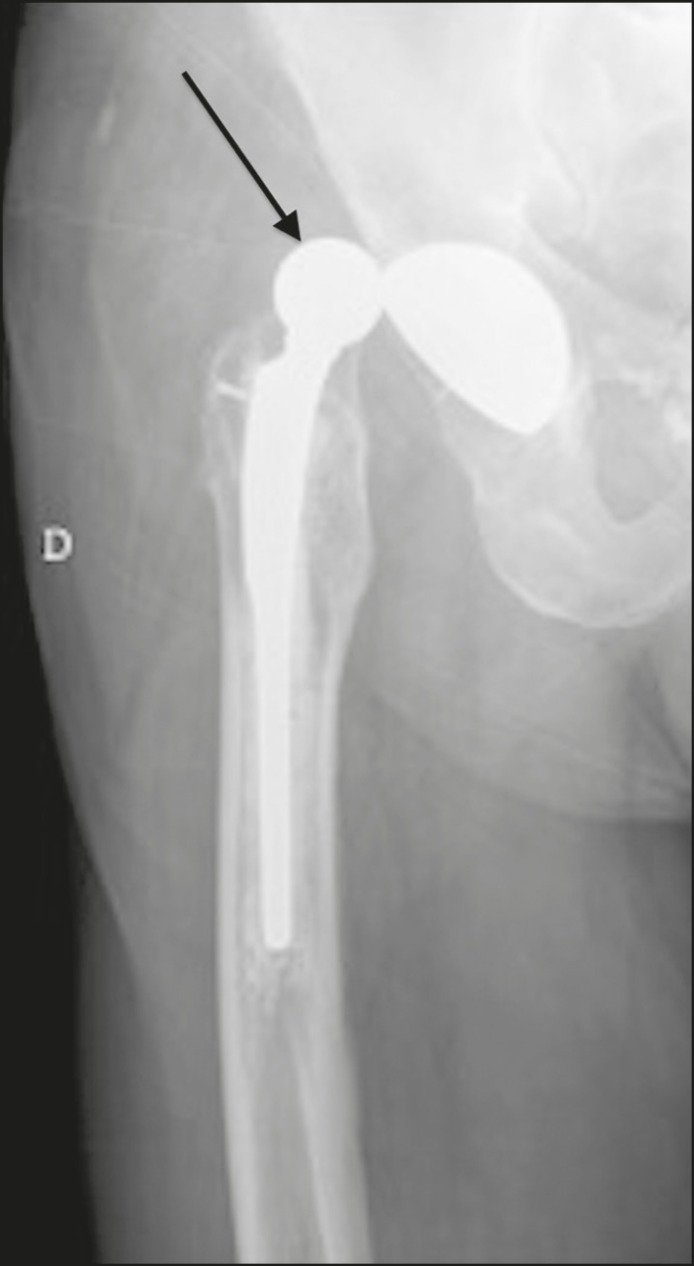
Anteroposterior X-ray of the right hip. Posterosuperior dislocation of the femoral component of right hip prosthesis (arrow), five years after the surgical procedure.
